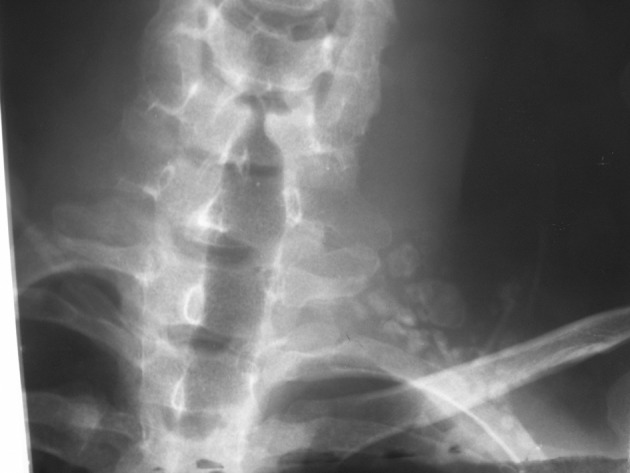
Chest X-ray of the patient shows a well-defined salient osseous expansion with spongy-form sclerotic areas on the posterolateral side of the first left rib.
